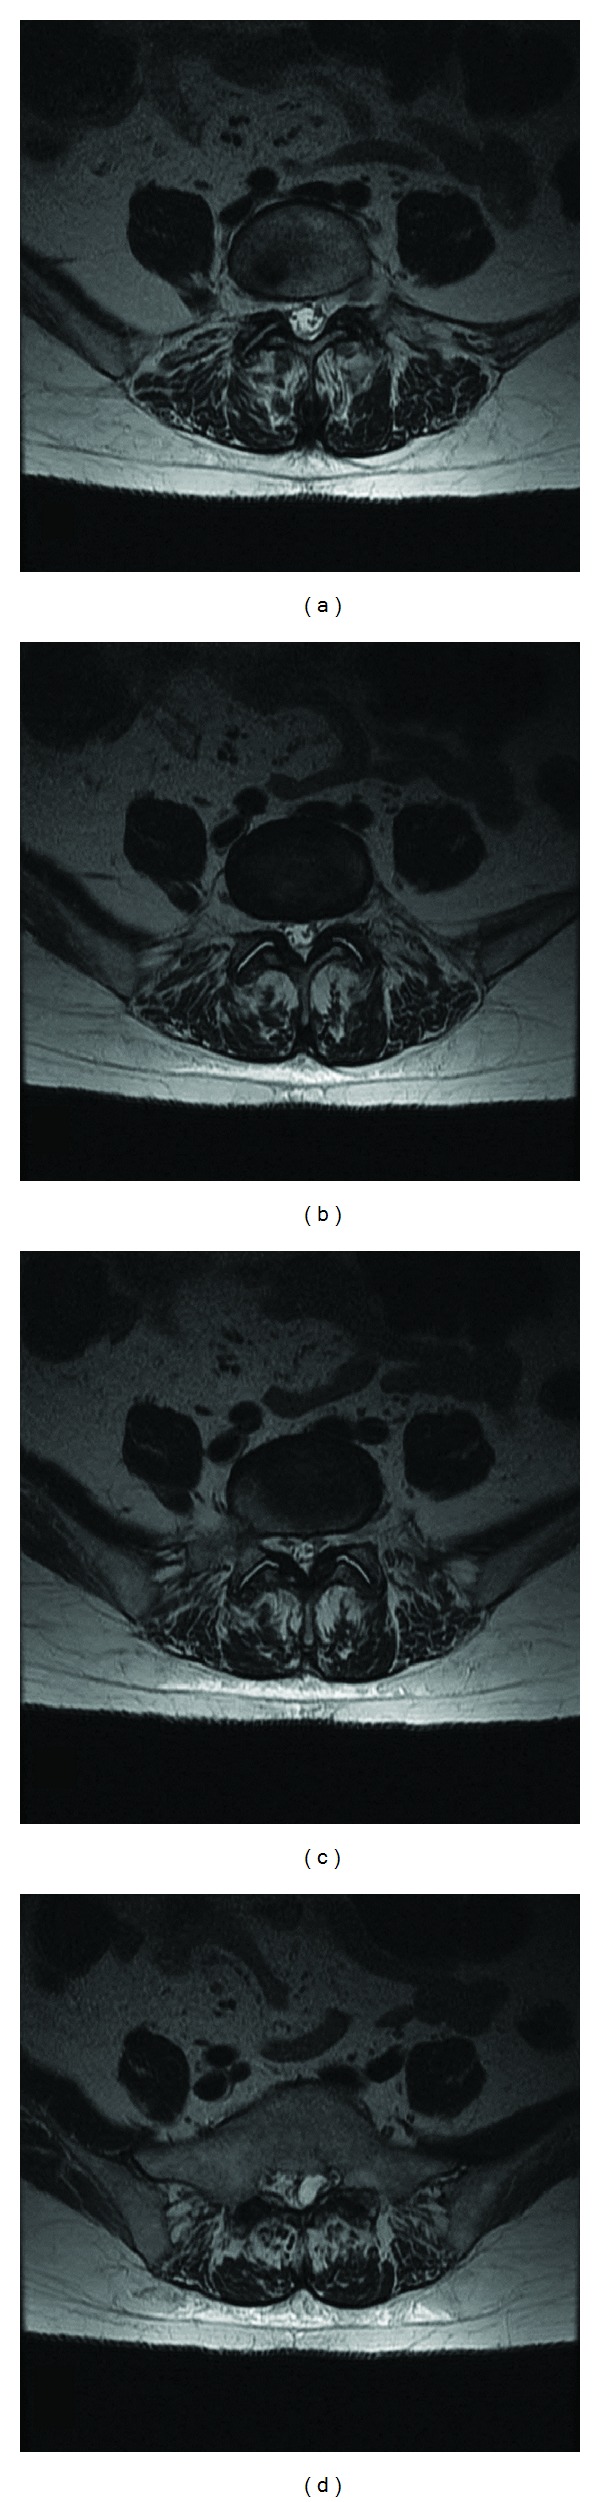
Axial T2-weighted MRI images showing sequential cuts ((a)–(d)) inferiorly from the L5-S1 facet joint showing an intrasacral mass separate from the L5-S1 joint.
